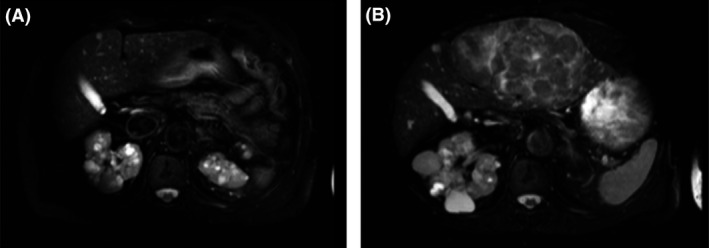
Findings of the T2‐weighted MRI. (A) Two years previously, liver MRI was normal. (B) The whole left hepatic lobe was deformed due to a heterogeneous tumor. No lymph node enlargement or ascites were observed.
